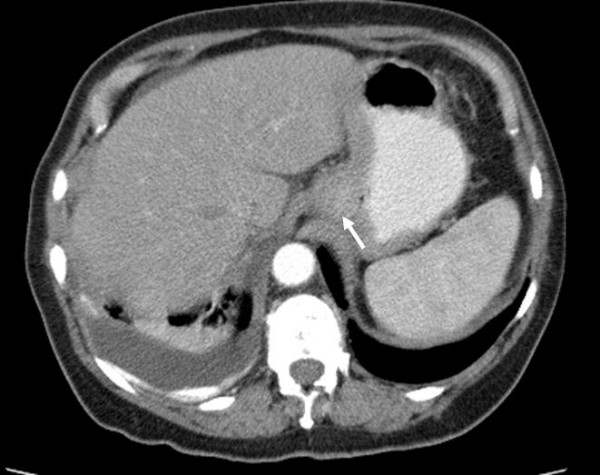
CT abdomen with oral contrast in patient 2 demonstrating thickening below the oesophagogastric junction (indicated by arrow) and residual right sided pleural effusion.
